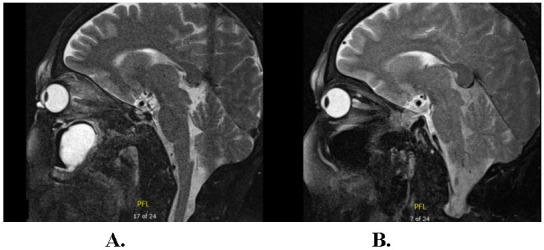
MRI with contrast, sagittal view. A. Enlargement of left extraocular muscles with enlargement of their tendon. A maxillary retention cyst was also noted. B. Normal size of right eye extraocular muscles
